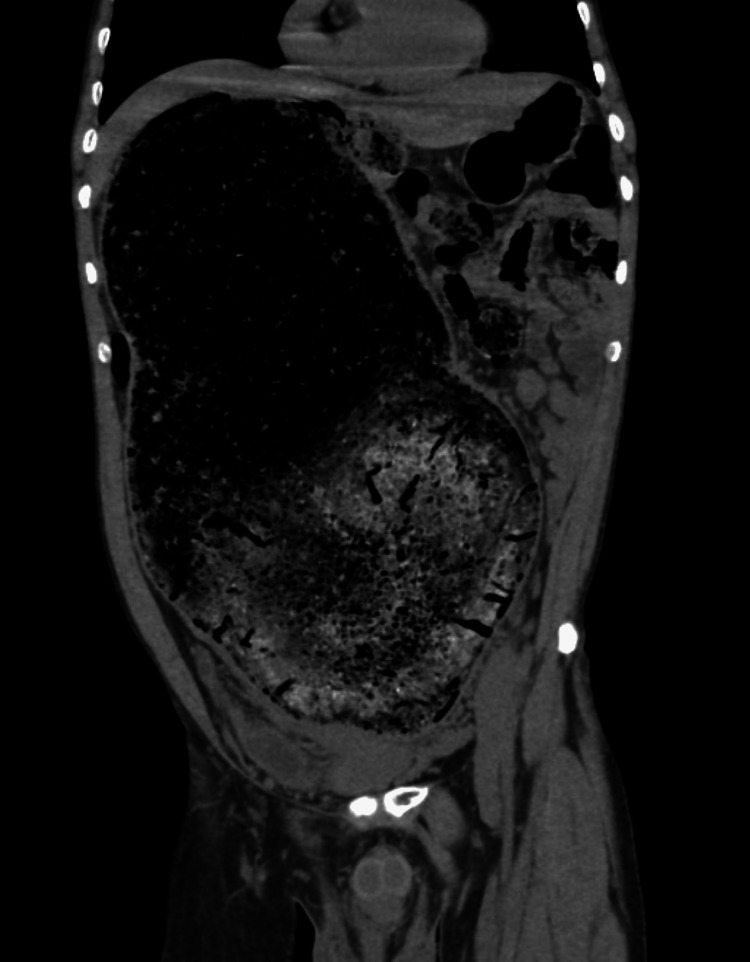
Coronal CT demonstrating sigmoid fecaloma. Coronal CT reconstruction demonstrating significant dilation of the proximal colon upstream from an obstructing fecaloma measuring 40 cm in length and 25 cm in width, without evidence of free air or perforation. CT: computed tomography
